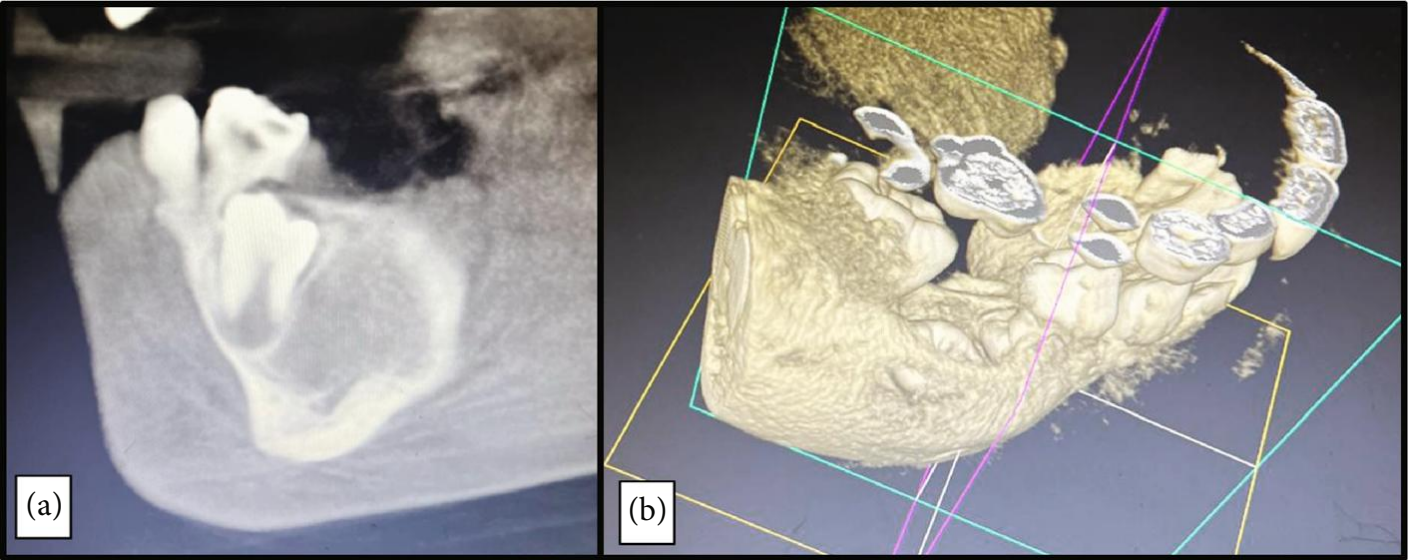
(a) Postoperative CBCT after 3 months and (b) 3D reconstructed image during the same period.
